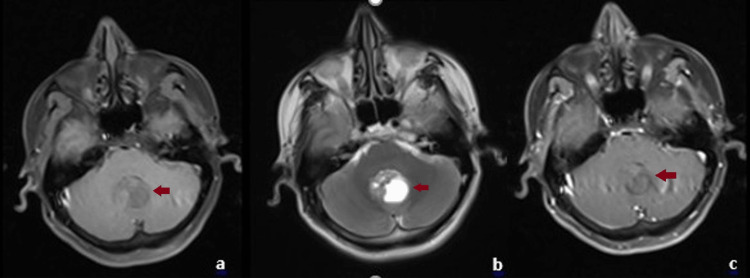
Axial magnetic resonance imaging (MRI) scans of the posterior fossa lesion (a) T1-weighted image showing a hypointense mass in the midline cerebellum. (b) T2-weighted image demonstrating a hyperintense signal with a cystic component. (c) Contrast-enhanced T1-weighted image revealing heterogeneous enhancement of the solid portion.
